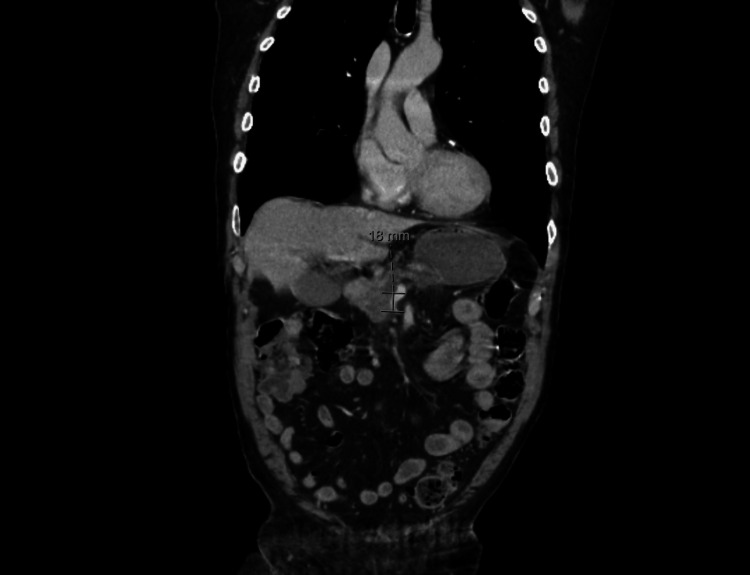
CT of the abdomen and pelvis showing a 4 cm mass on the pancreatic head abutting the superior mesenteric vein.
